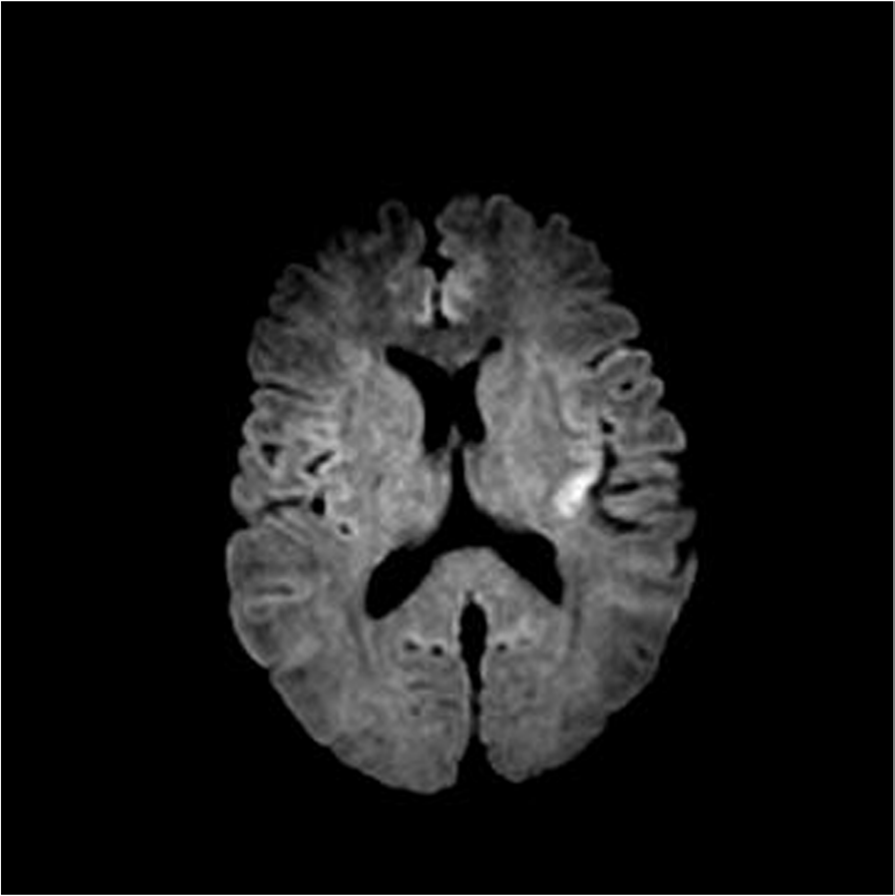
Magnetic resonance imaging of the brain demonstrated focal diffusion-restricted lesions in the left insular lobe and left centrum semiovale, suggesting seizure-related changes rather than acute infarction
